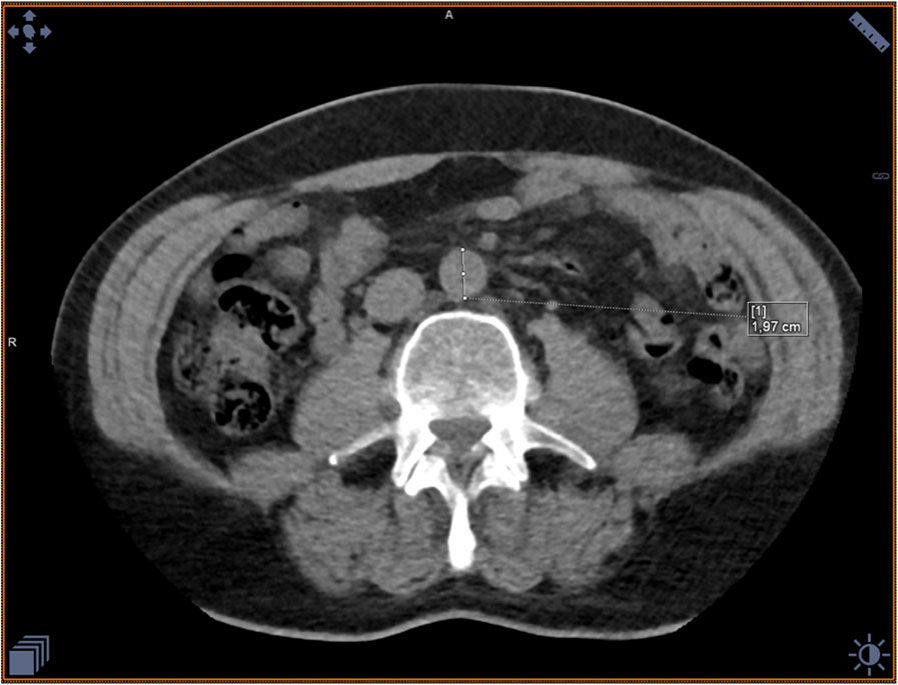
Transverse measurement done with CT was 19.7 mm, US grossly overestimated this at 44.6 mm. It should be mentioned that the participant in question was obese, making US examination troubling
